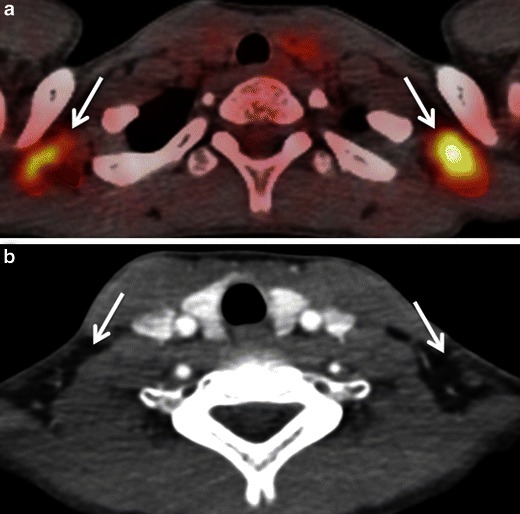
a Axial PET/CT image depicts bilateral symmetrical areas of avid FDG uptake in the supraclavicular regions (*arrows*). These may be confused for metastatic supraclavicular adenopathy. b Corresponding axial CECT image detects no focal abnormality in the supraclavicular regions (*arrows*). Note that the areas of high uptake seen in a correspond to low attenuation fatty tissue (*arrows*) in b
